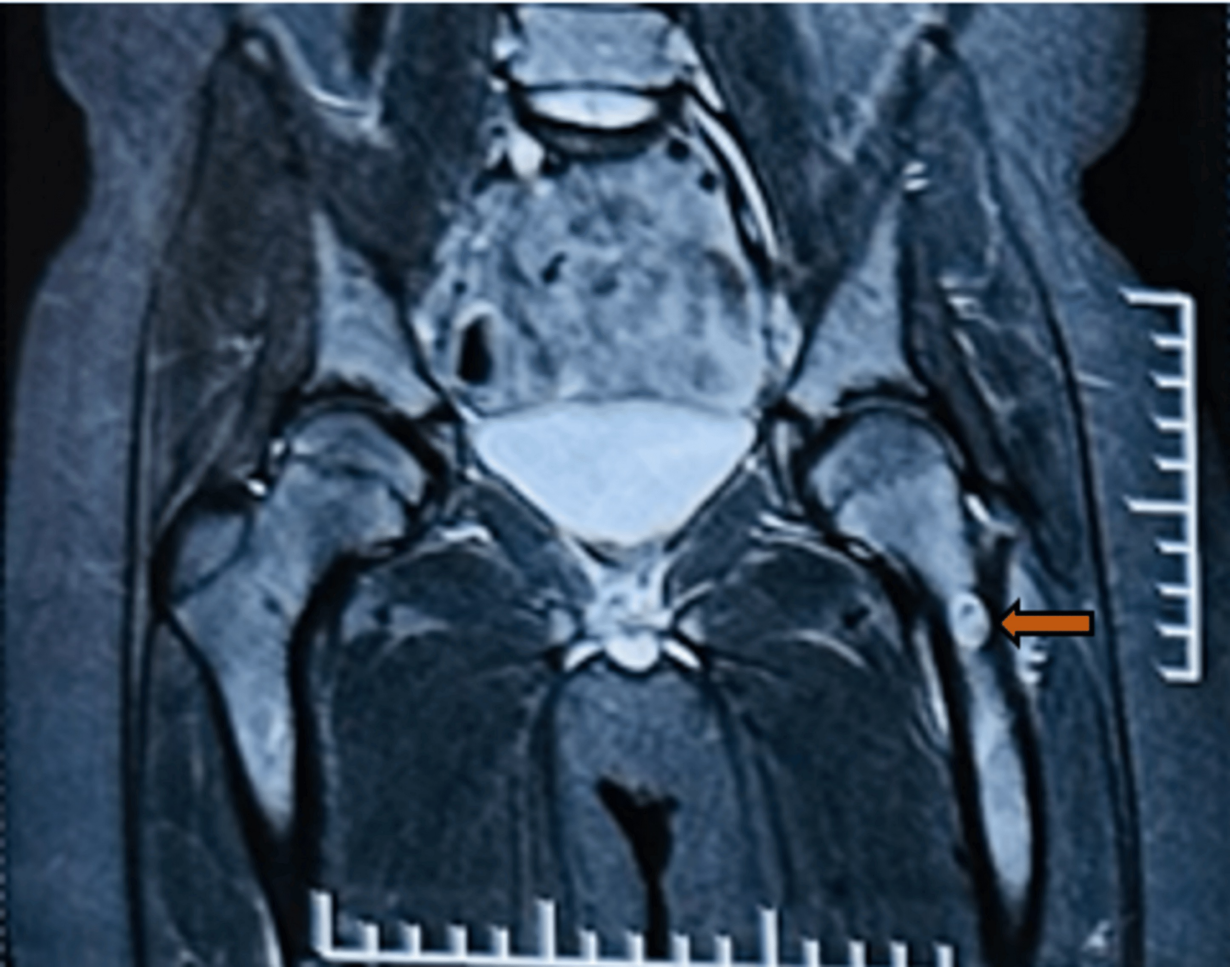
Magnetic resonance imaging of the hip, coronal section showing oval hyperintense lesion in the neck of left femur suggesting the possibility of osteoid osteoma (Orange arrow)
